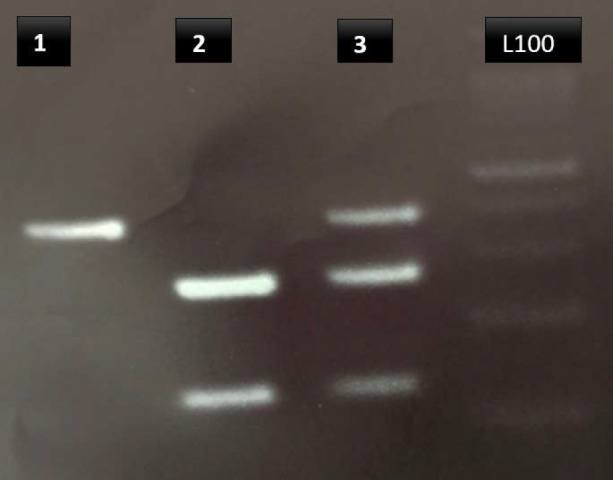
Digestion RFLP rs2241880 withe BfuI Enzyme: 1= CC, 386bp - 2= TT, 266bp-120bp - 3= CT, 386bp- 266bp-120bp
